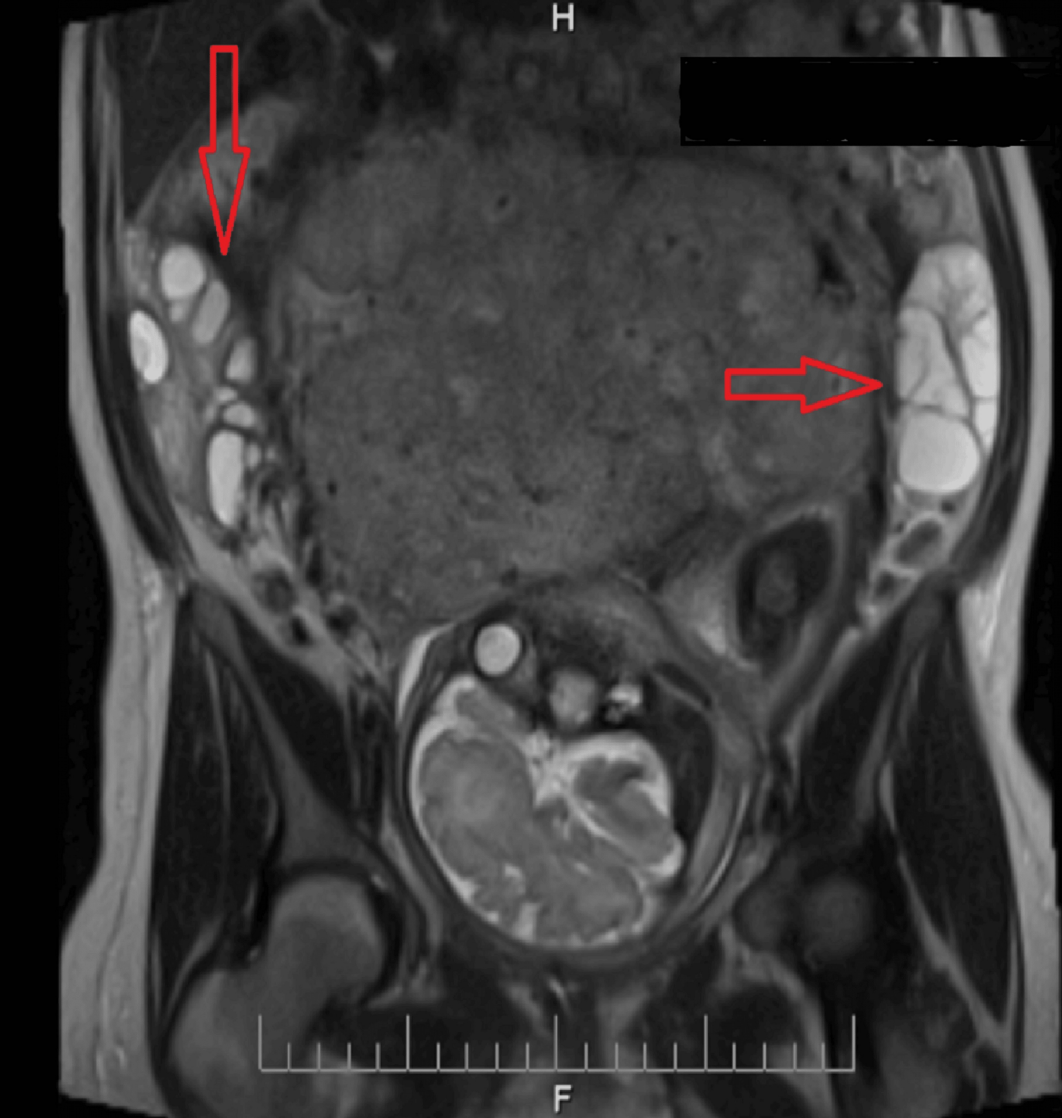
MRI image of enlarged ovaries H: head; F: foot
